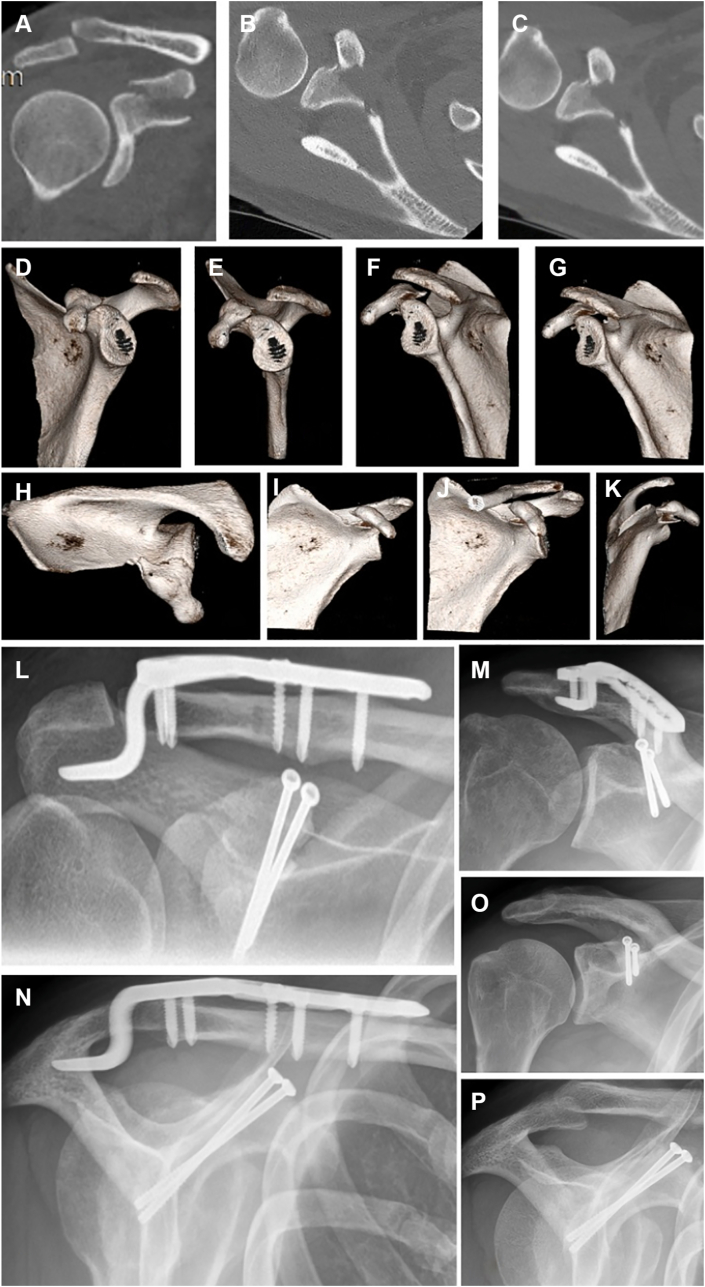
Double disruption injury of SSSC and treatment with hook plate and screws. A fracture of the coracoid process with acromioclavicular joint separation of the right shoulder occurred in a 32-year-old male treated with open reduction of the AC joint and implantation of a hook plate, along with closed reduction of the coracoid process and fixation using 2 screws; the hook plate was removed after 3 months. (A–K) 2- and 3-dimensional CT images demonstrating a double disruption of the SSSC of the right shoulder, including a fracture of the coracoid process with ACJ separation of the right shoulder. (L–N) Immediate post-operative radiograph (L) and post-operative radiographs obtained 3 months after open reduction of the ACJ and implantation of a 15-mm 6-hole hook plate, along with closed reduction of the coracoid process and fixation using 2 3.5 mm screws. (O–P) Radiographs at 3-month follow-up showing complete fracture union, proper alignment, and absence of implant-related complications following removal of the hook plate. In the case of coracoid process fracture combined with ACJ separation, fixation of both the ACJ and coracoid process was selected to restore the clavicle–scapula linkage and prevent persistent ring instability. Open reduction of the ACJ with implantation of a 15-mm 6-hole hook plate, together with closed reduction of the coracoid process and fixation using 2 3.5-mm screws, achieved complete fracture union, proper alignment, and no implant-related complications at 3-month follow-up after hook plate removal. *SSSC*, superior shoulder suspensory complex; *ACJ*, acromioclavicular joint; *CT*, computed tomography.
